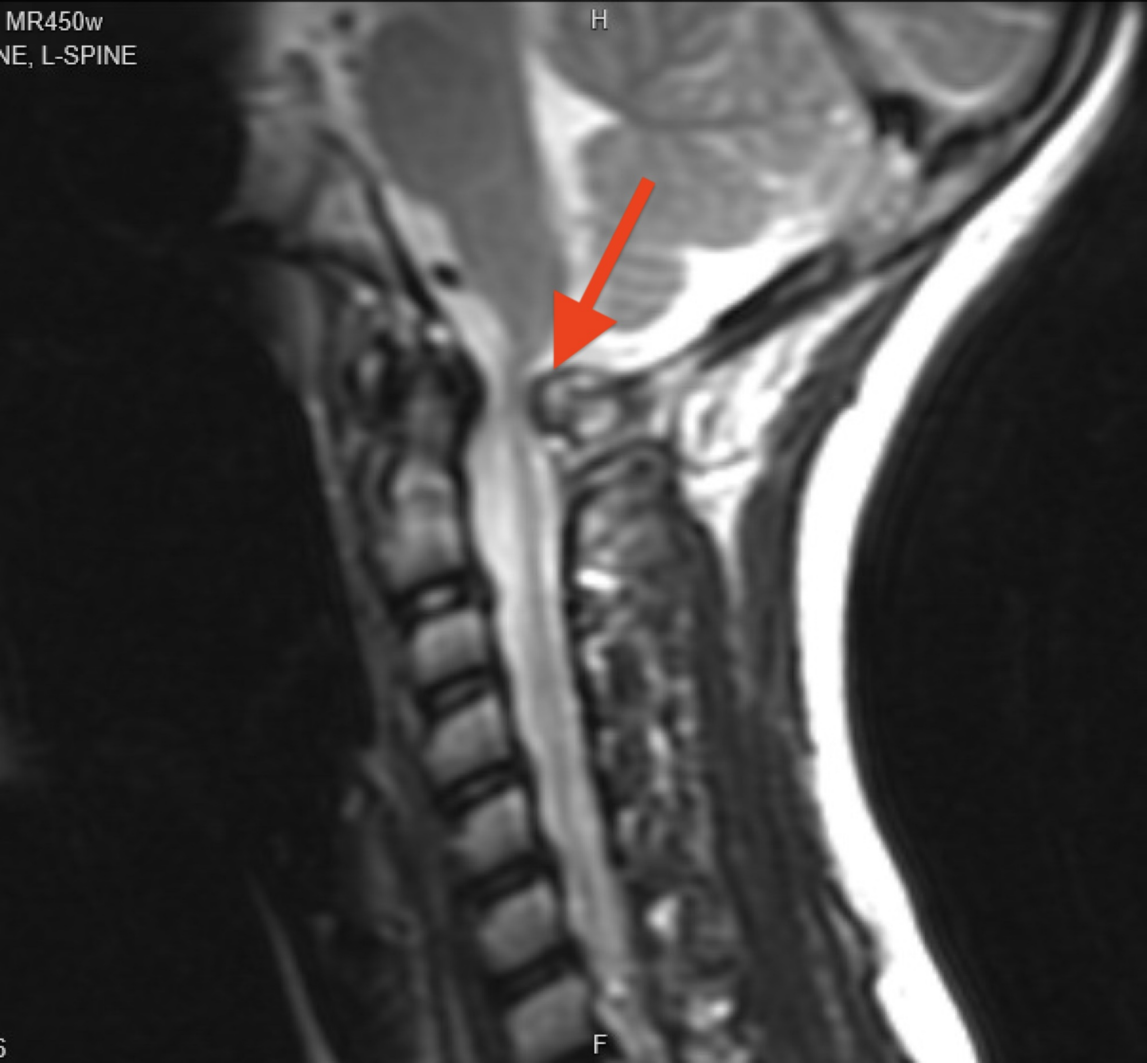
Cervical spine MRI sagittal view demonstrating an osteochondroma extending from the posterior arch of C1 and compressing the spinal cord
